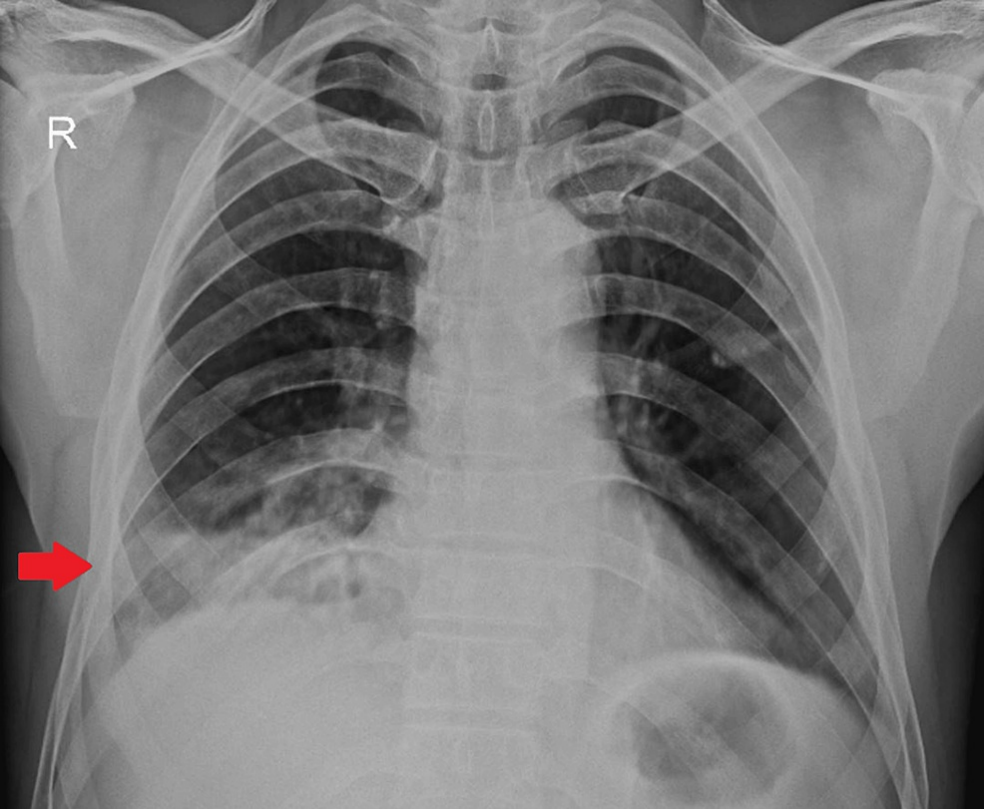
Chest X-ray posteroanterior view showing haziness in the right lower zone (red arrow)
